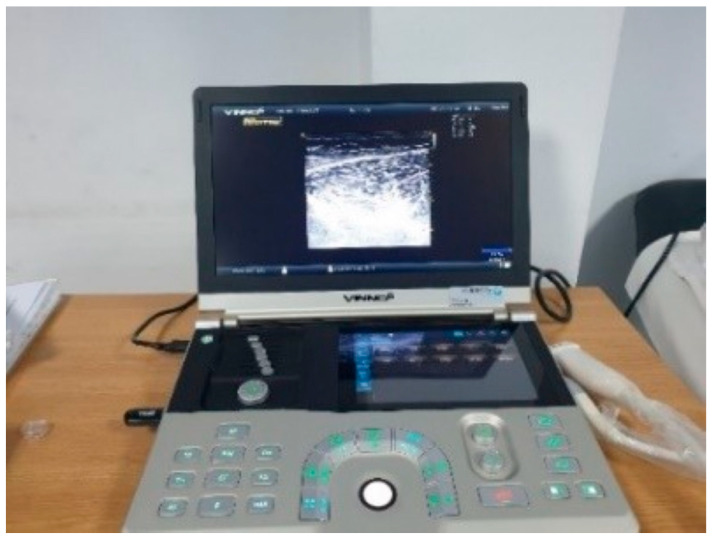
Ultrasound machine VINNO 6.
